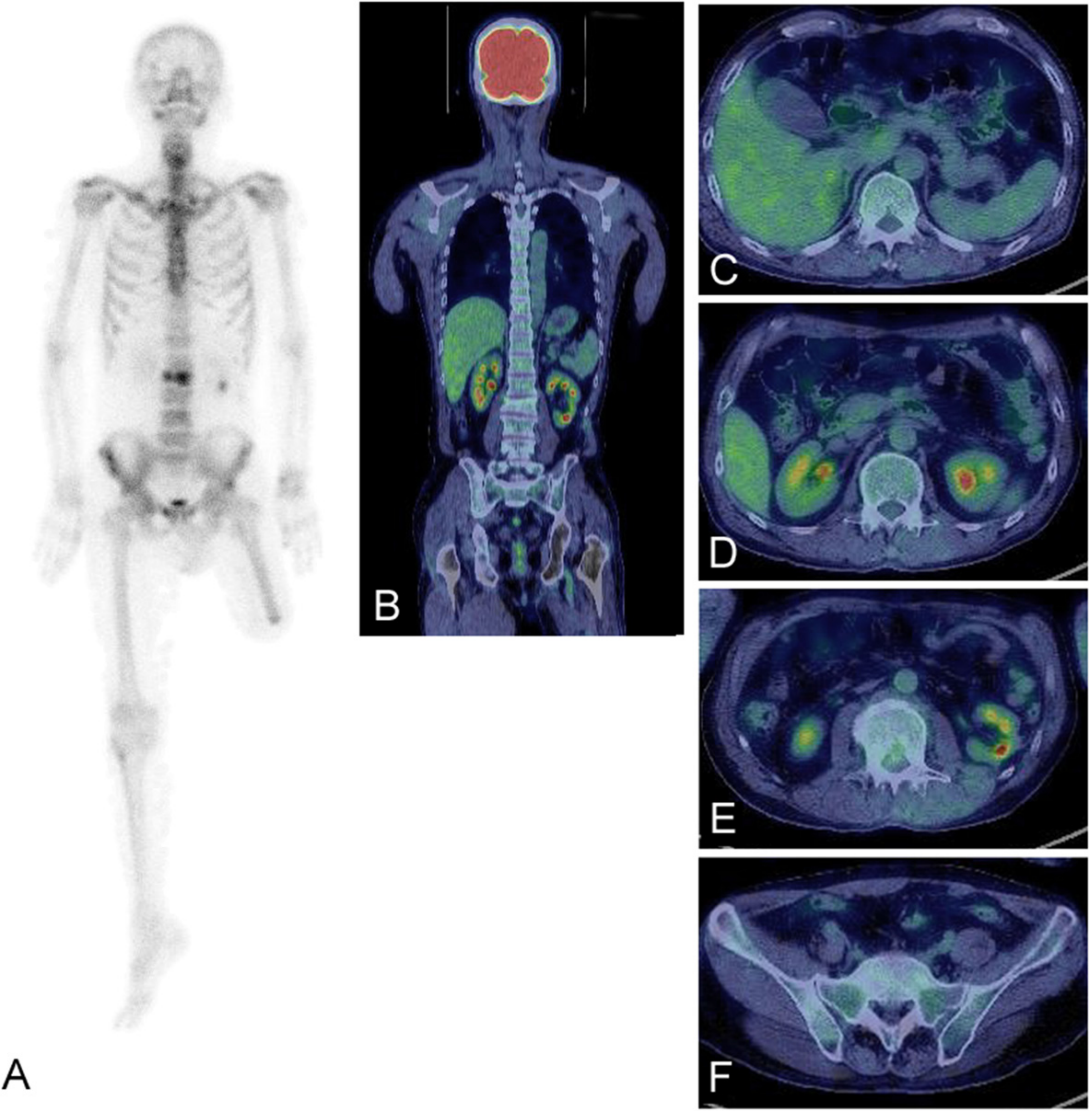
Positive findings can be seen on the bone scan in the fractured second lumbar vertebra (L2)(A). Coronal section (B) and axial sections of twelfth thoracic vertebra (T12) (C), L1 (D), L2 (E) and the first sacral vertebra (S1) (F) in FDG-PET are shown. There are no obvious differences between FDG uptake in metastatic vertebrae of T12 (C), L2 (E), and the non-metastatic vertebrae of L1 (D) and S1 (F). FDG-PET, [18F]-2-fluoro-2-deoxy-D-glucose-positron emission tomography.
